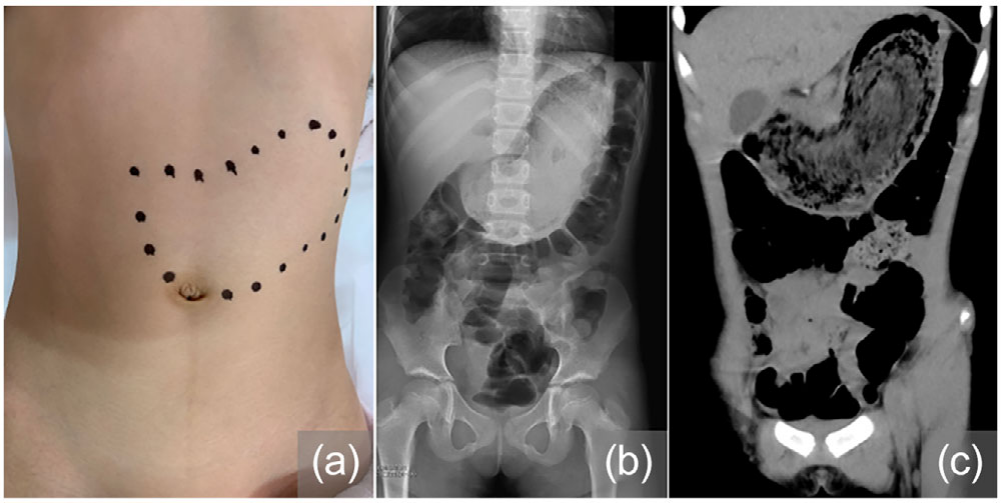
(a) A huge mass is palpated in the upper right abdomen. (b, c) X‐ray and abdominal computed tomography show a large gastric mass.
